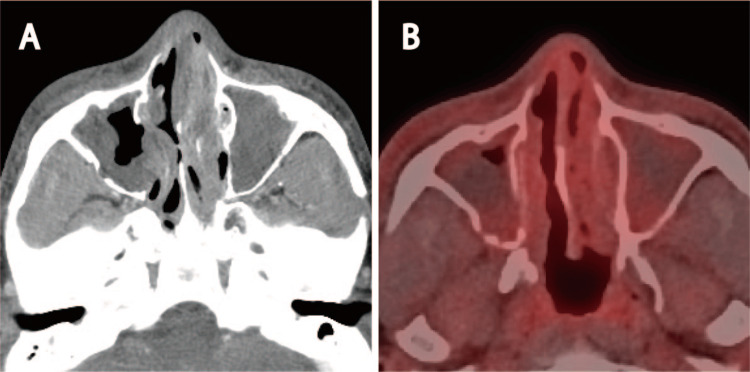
Post-treatment imaging findings (A) Computed tomography (CT) findings after chemoradiation therapy. The primary tumor has shrunk. Shadows are seen at the maxillary and ethmoidal sinuses. (B) Positron emission tomography-CT findings after chemoradiation therapy. The nasal soft tissue shadow has shrunk, and no abnormal hyperaccumulation can be identified. Weak accumulation (maximum standardized uptake value: 2.1) can be observed in the ethmoid and maxillary sinuses, which can be considered to indicate an inflammatory change after treatment.
